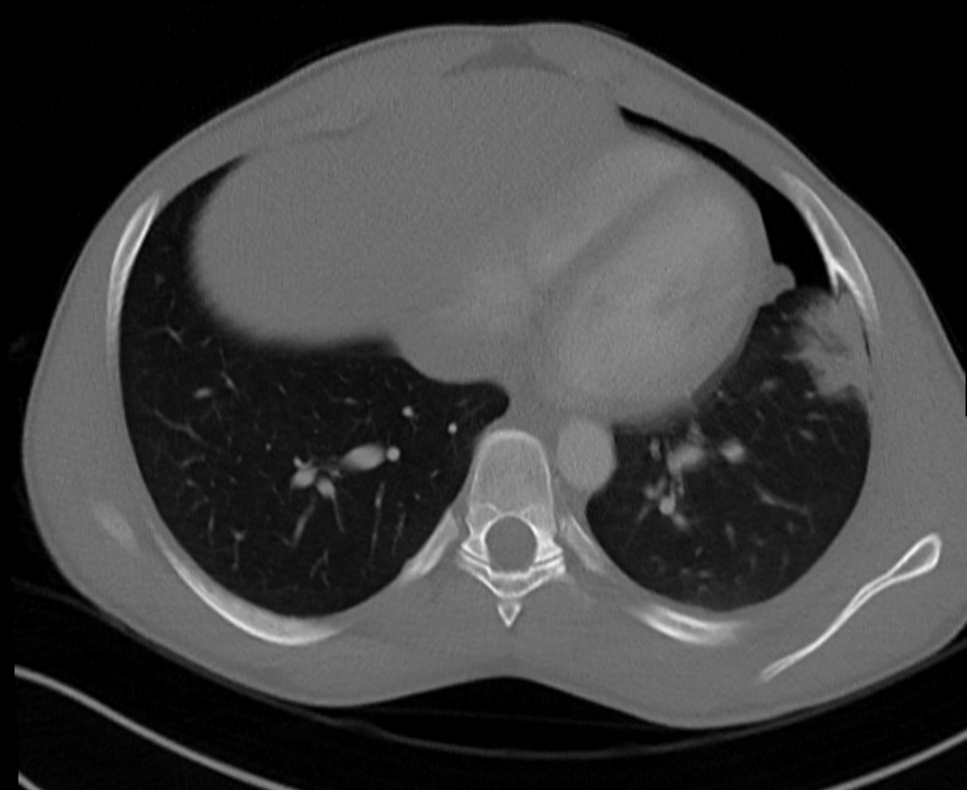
Axial CT angiography image (5 mm reconstruction; bone setting) showing osseous continuity of the osteochondroma and the sixth rib on the left side.
